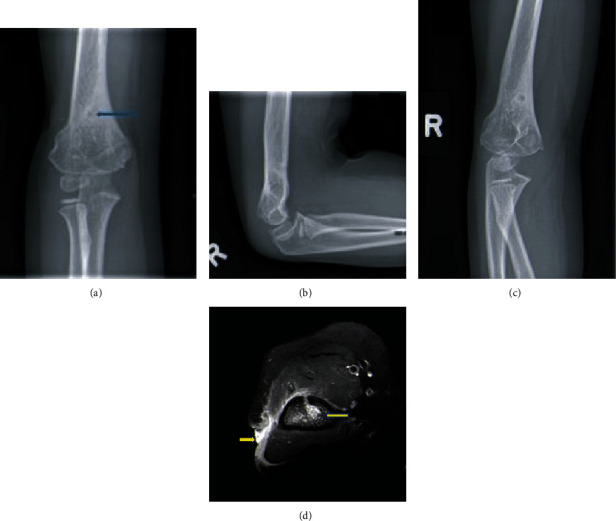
(a–c) AP, lateral, and oblique MRI of the right elbow with a subtle area of lucency (arrows) in the metaphysis. (d) Axial T1 postcontrast MRI with an enhancing lesion (large arrow) in the bone with anterior cortical disruption and enhancement anterior to the humerus extending to the lateral sinus tract (small arrow).
